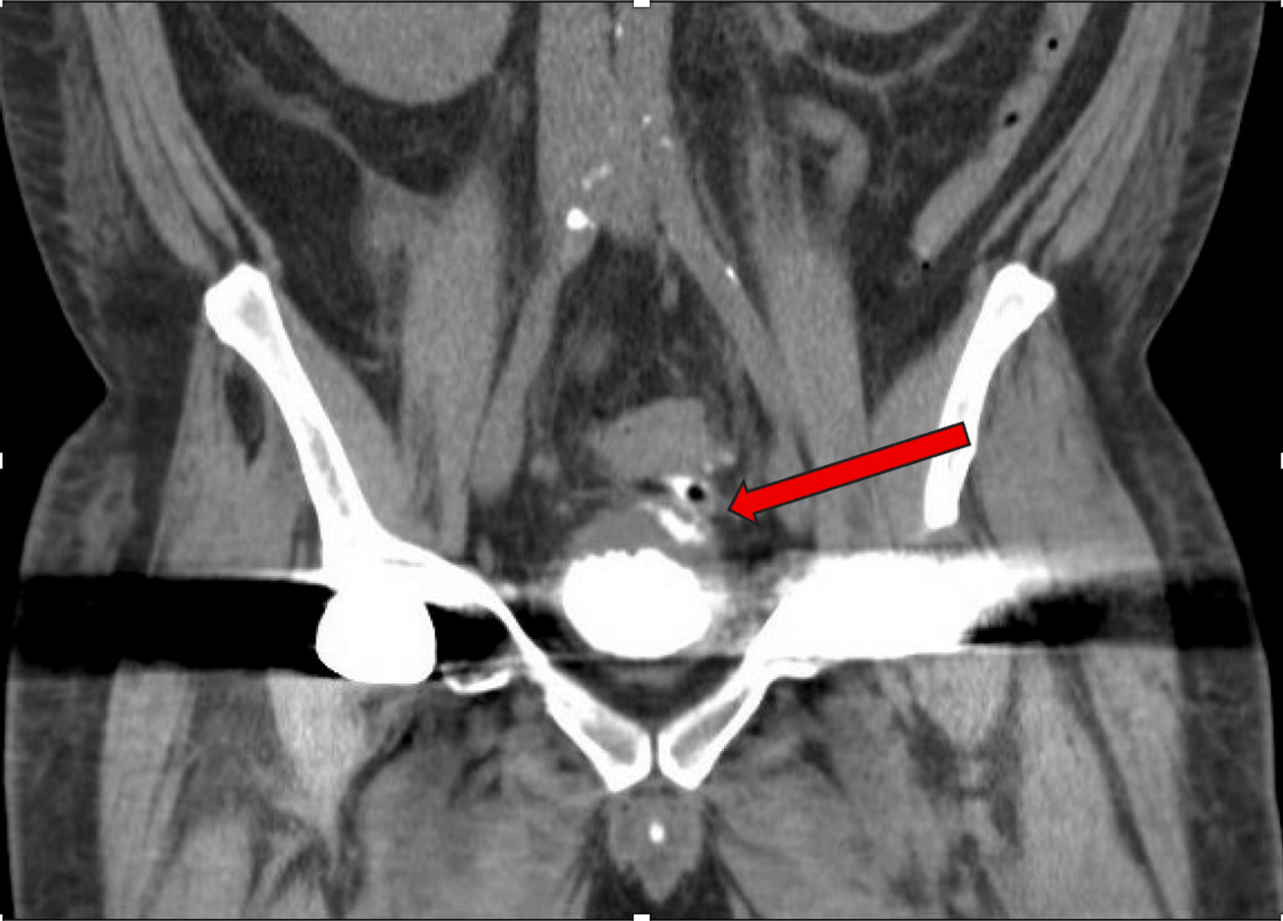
CT pelvis cystogram with contrast Contrast is seen traveling from the bladder to the colon (red arrow)
